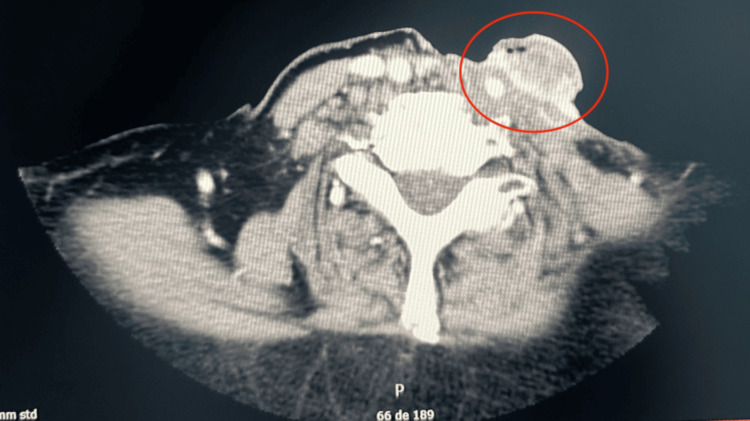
Axial contrast-enhanced cervical CT (August 2019) demonstrating an ulcerated cutaneous lesion of the left anterolateral neck (greatest dimension ~35 mm), consistent with locoregional recurrence CT: computed tomography
